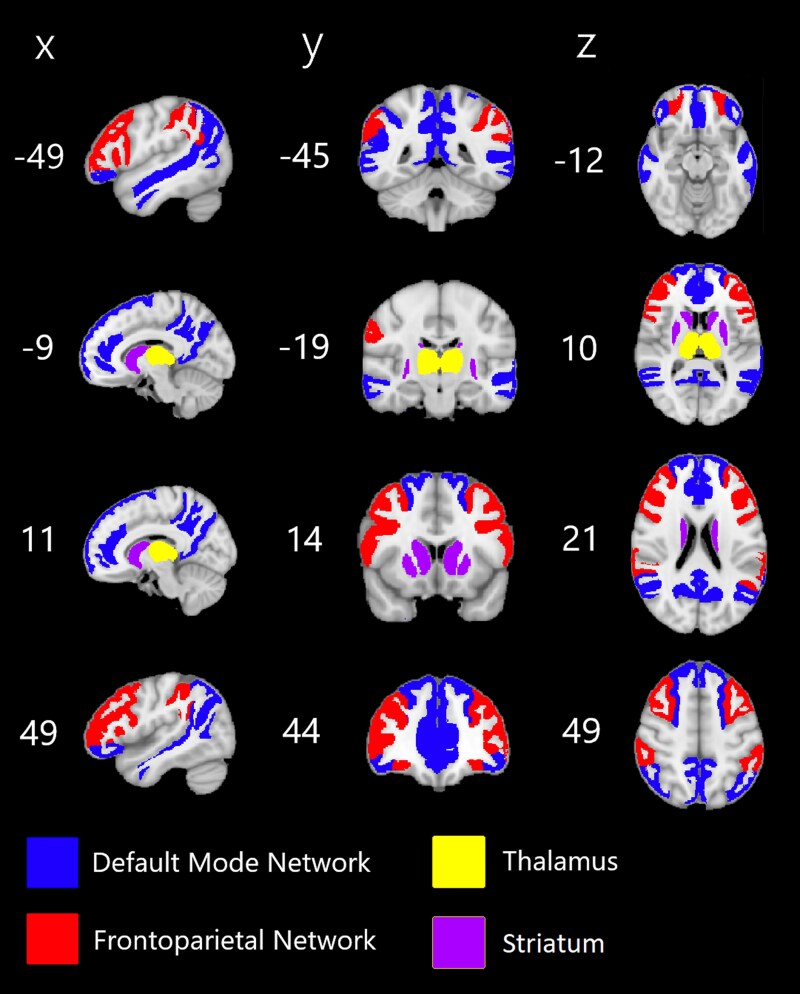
Overview of ROIs considered in this study. ROIs are extracted from a SLANT brain segmentation of the Montreal Neurological Institute (MNI) T1-weighted image. Columns show sagittal, coronal and axial slices with corresponding MNI coordinates, respectively.
